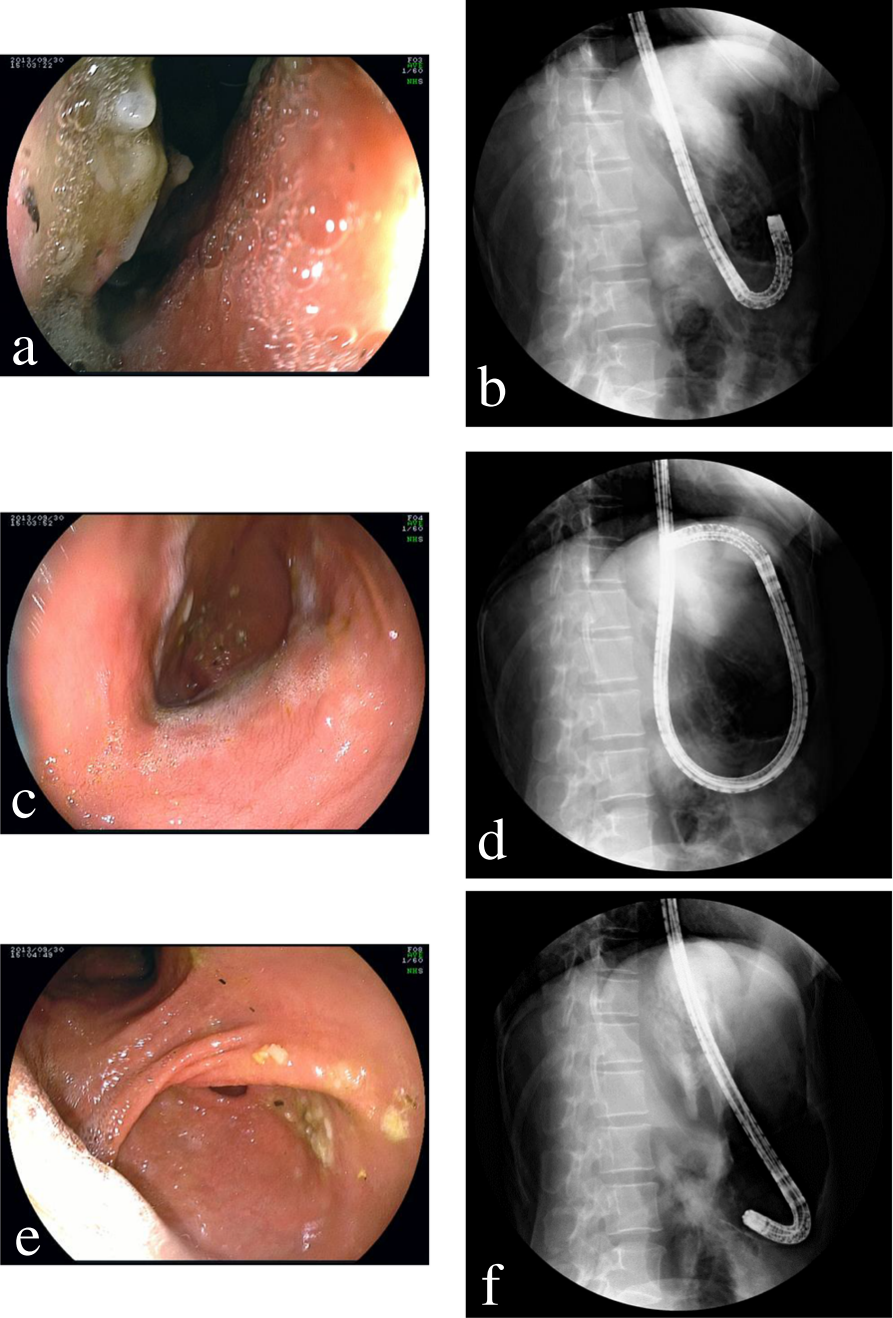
Endoscopic reduction of gastric volvulus using radiographic imaging. a Upper GI endoscopy shows the dilated stomach containing residual food and fluid (GI image of a is b). c The endoscope cannot be passed through the pylorus (GI image of c is d). e With various endoscopic maneuvers, such as clockwise rotation and pulling the GI endoscope back, the gastric volvulus is reduced and the endoscope passes through the pylorus into the duodenum (GI image of e is f). *GI* gastrointestinal
